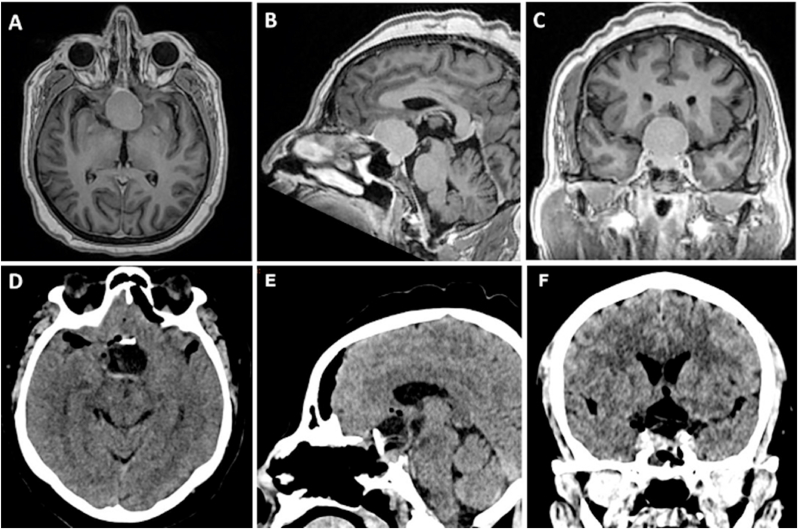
Preoperative MRI: (A) axial, (B) sagittal, and (C) coronal views from a T1WI C+ showed a dorsally projected lesion compatible with a TSM. Postoperative CT scan: (D) axial, (E) sagittal, and (F) coronal views in which gross total resection is observed.
